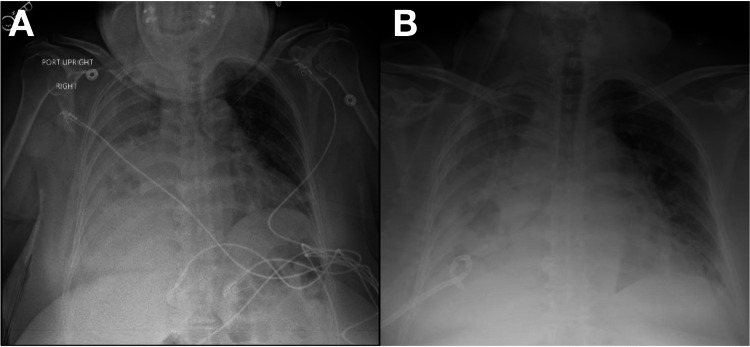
X-ray of the chest before and after right intrapleural chest tube placement. (A) AP X-ray of the chest frontal view on the day of admission demonstrates large right-sided pleural and parenchymal opacities, consistent with pleural fluid and associated volume loss/pneumonia. (B) AP X-ray of the chest frontal view on the second day of admission and interval placement of right pleural drain shows partial improvement in expansion of the right lung, which is still significantly hypoinflated. AP, anteroposterior
